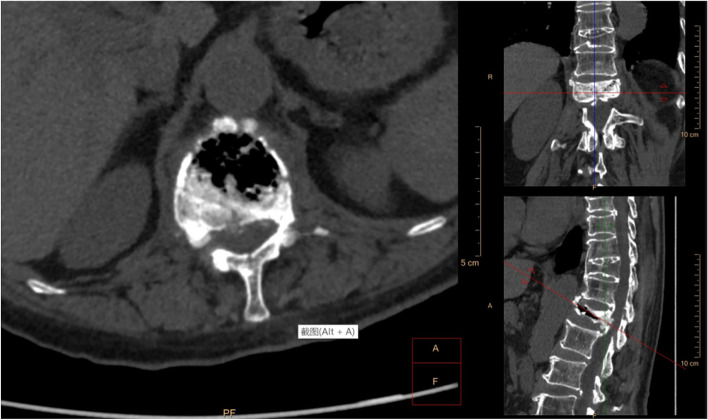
Sagittal, coronal and horizontal three-dimensional CT images of T12 vertebral body in patients with osteoporosis and Kummell's disease
